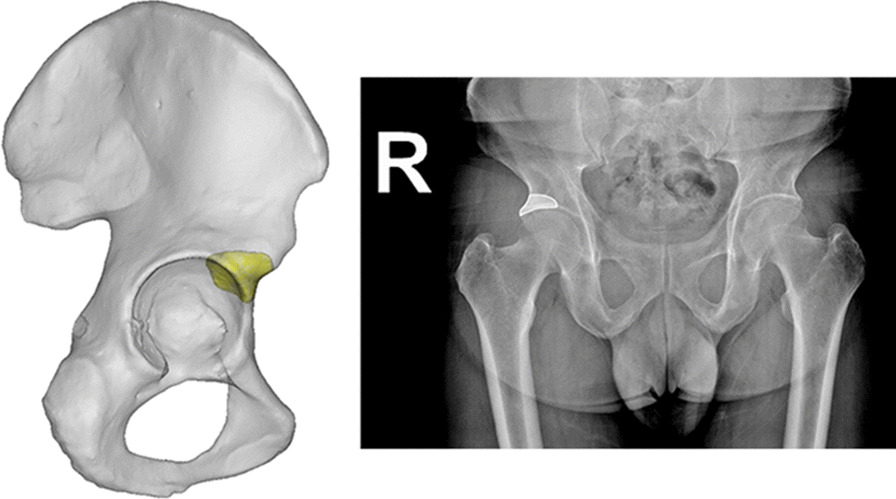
X-ray of the anterosuperior bone stock of the acetabulum
